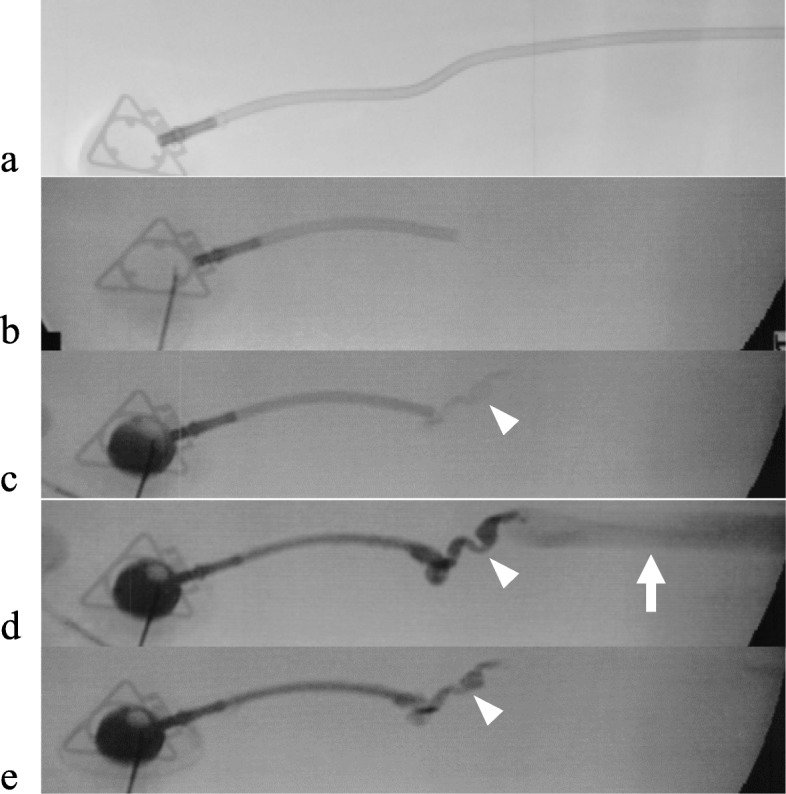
Contrast injection via totally implantable venous access port (TIVAP) with fractured catheter. a X-ray photography obtained after implantation of TIVAP. b The fractured catheter was spontaneously found 7 months after implantation of TIVAP. c, d, e Saline was injected upon removal of the TIVAP septum; back flow from the TIVAP septum was slightly bloody. When contrast-enhanced sodium was injected through the TIVAP septum, contrast flow was observed in the femoral vein (arrow). A narrow tract can be seen between the tip of the fractured catheter and the femoral vein (arrowhead)
